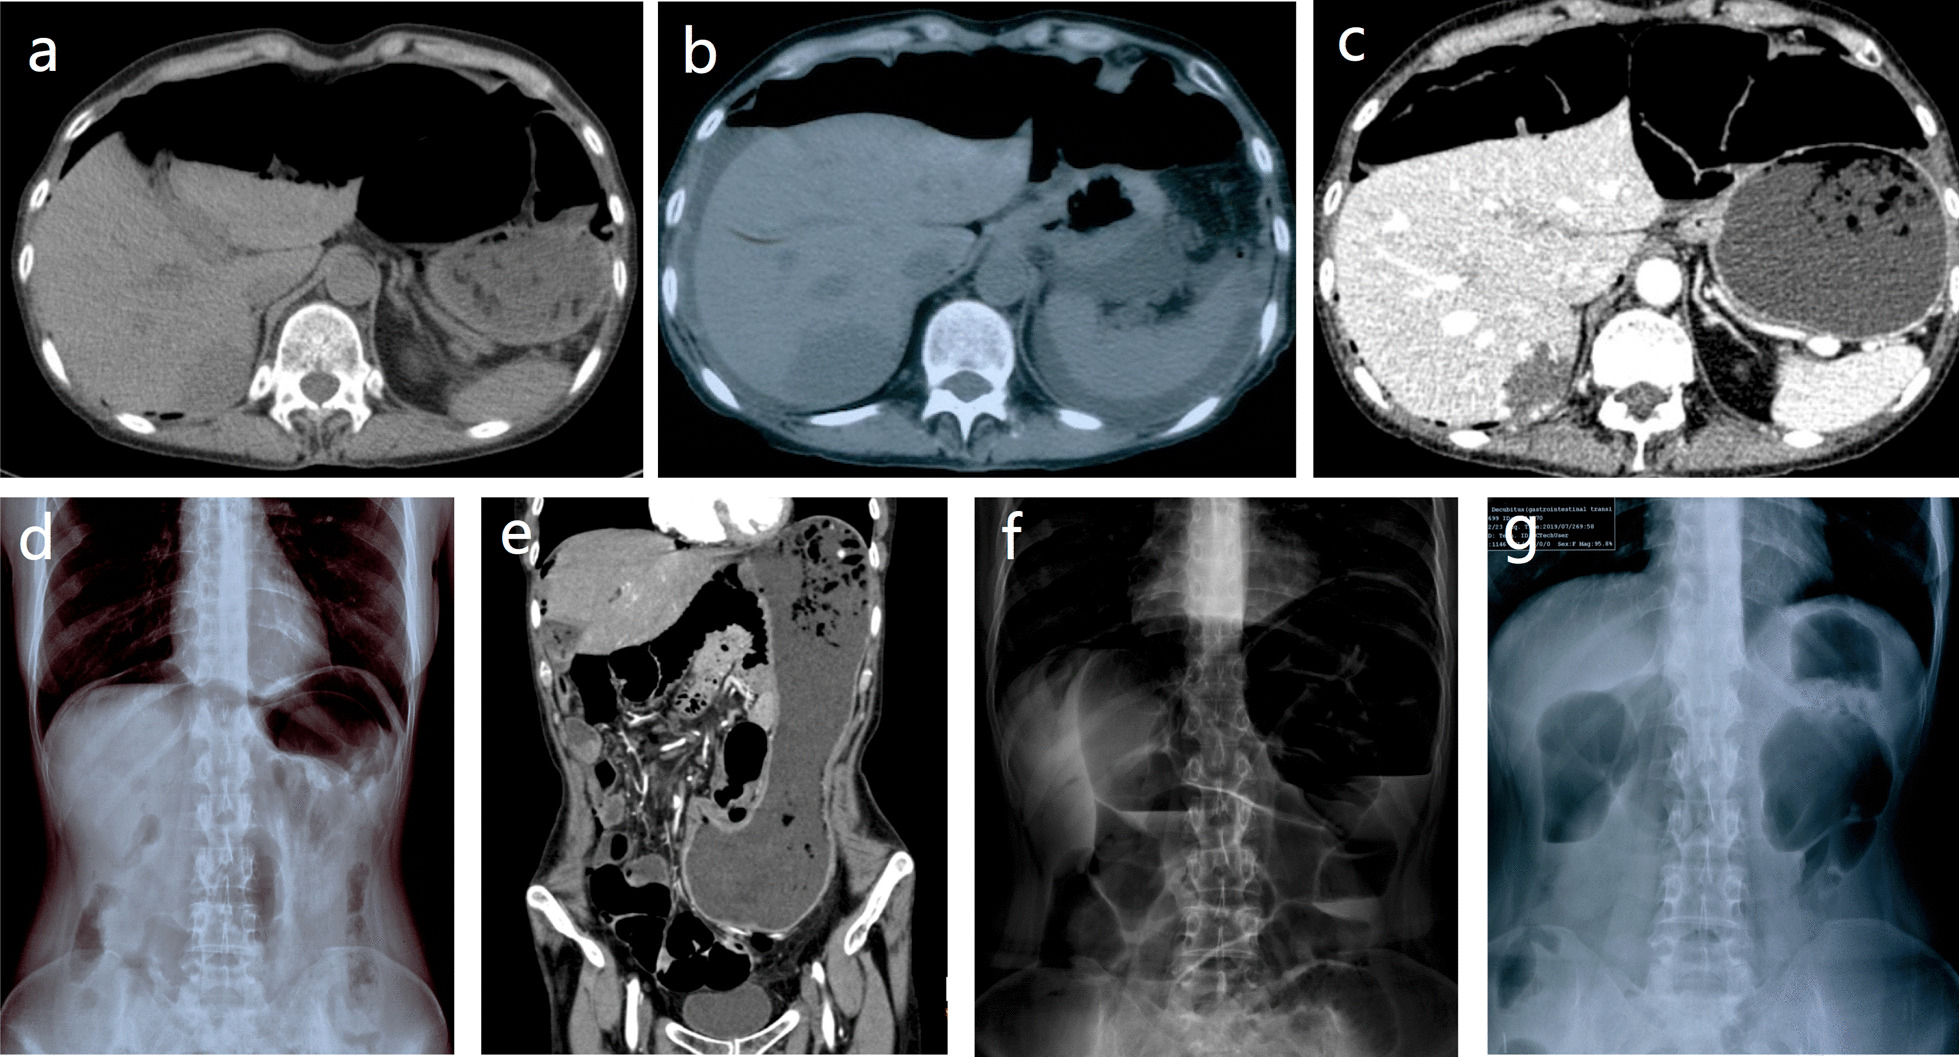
Radiography of abdominal cavity and intestine. a Apparently intestinal dilatation and free-air in abdominal cavity in abdominal CT scan. b CT scan showing free-air in the peritoneal cavity again with a small amount of perihepatic and perisplenic effusion. c Abdominal CT displaying subdiaphragmatic free-air again and intestinal dilatation with recurrence of the symptoms. d A translucent crescent or area (free air) below the diaphragm could be observed on upright posteroanterior chest radiography. e CT scan revealing marked distension of the stomach, which was vertical and occupied a low position. f The upright conventional abdominal radiography showing the presence of intestinal dilatation, air-fluid level formation. g Subdiaphragmatic free-air disappeared after gastrointestinal decompression in the upright abdominal radiography
